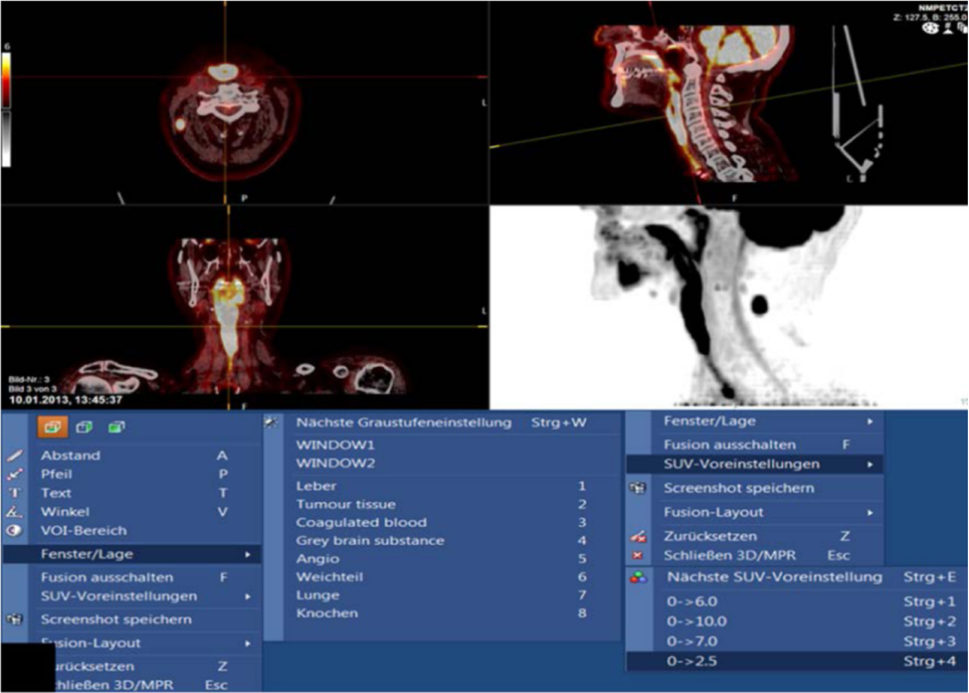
PACS system Sectra Workstation IDS7 Version 17.1.10 with NM Fusion image display allowing demonstration of the volumetric PET-CT data in all three planes and as MIP. The various preset window and SUV settings are shown in the bottom half. The PET images shows the long segment of avid enhancement of the neopharynx in a patient with tumour recurrence (same patient as in Fig. 8) and a lymph node metastasis on the right
